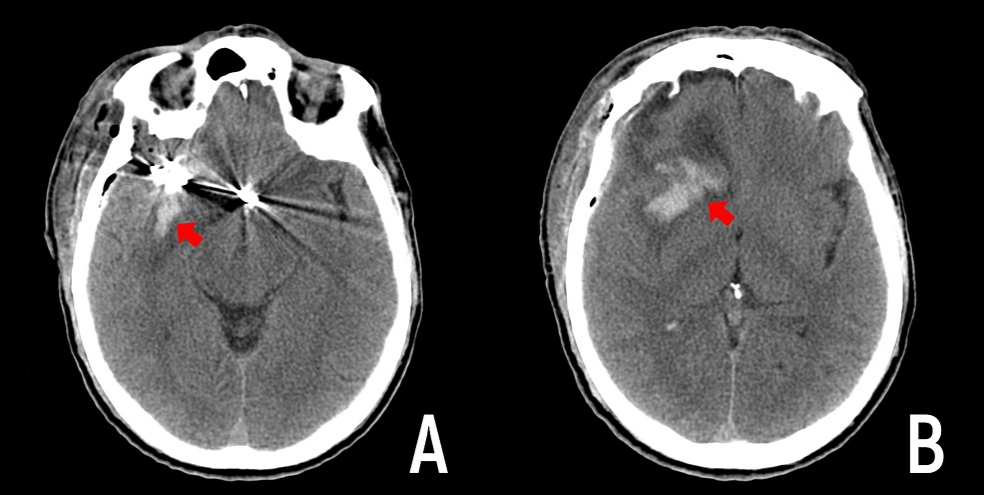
Hemorrhagic complication in the first case CT scan of the head on the first day after clipping of the aneurysms of the right middle cerebral artery and anterior communicating artery. A - Hemorrhagic imbibition is located along the Sylvian fissure with the transition to the right frontal lobe (red arrow). B - Hemorrhagic imbibition of the deep portions of the right frontal lobe with perifocal edema (red arrow).
